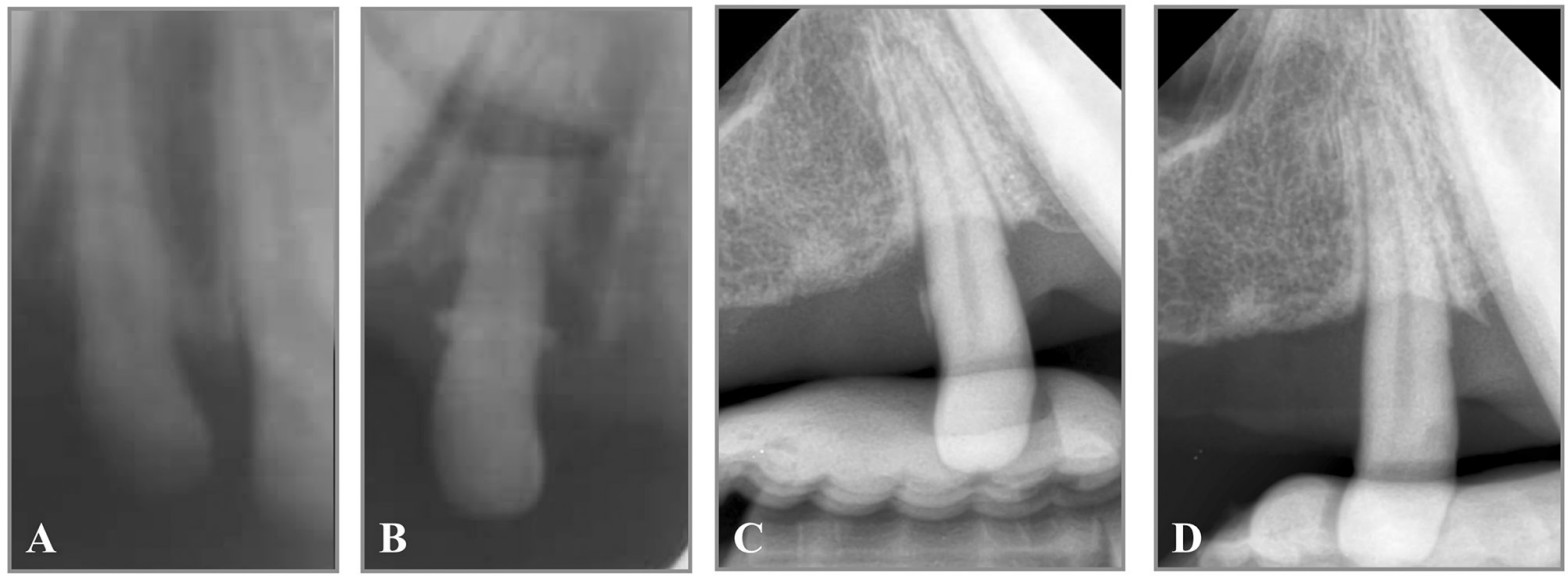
Radiographic analysis of the alveolar bone. (
